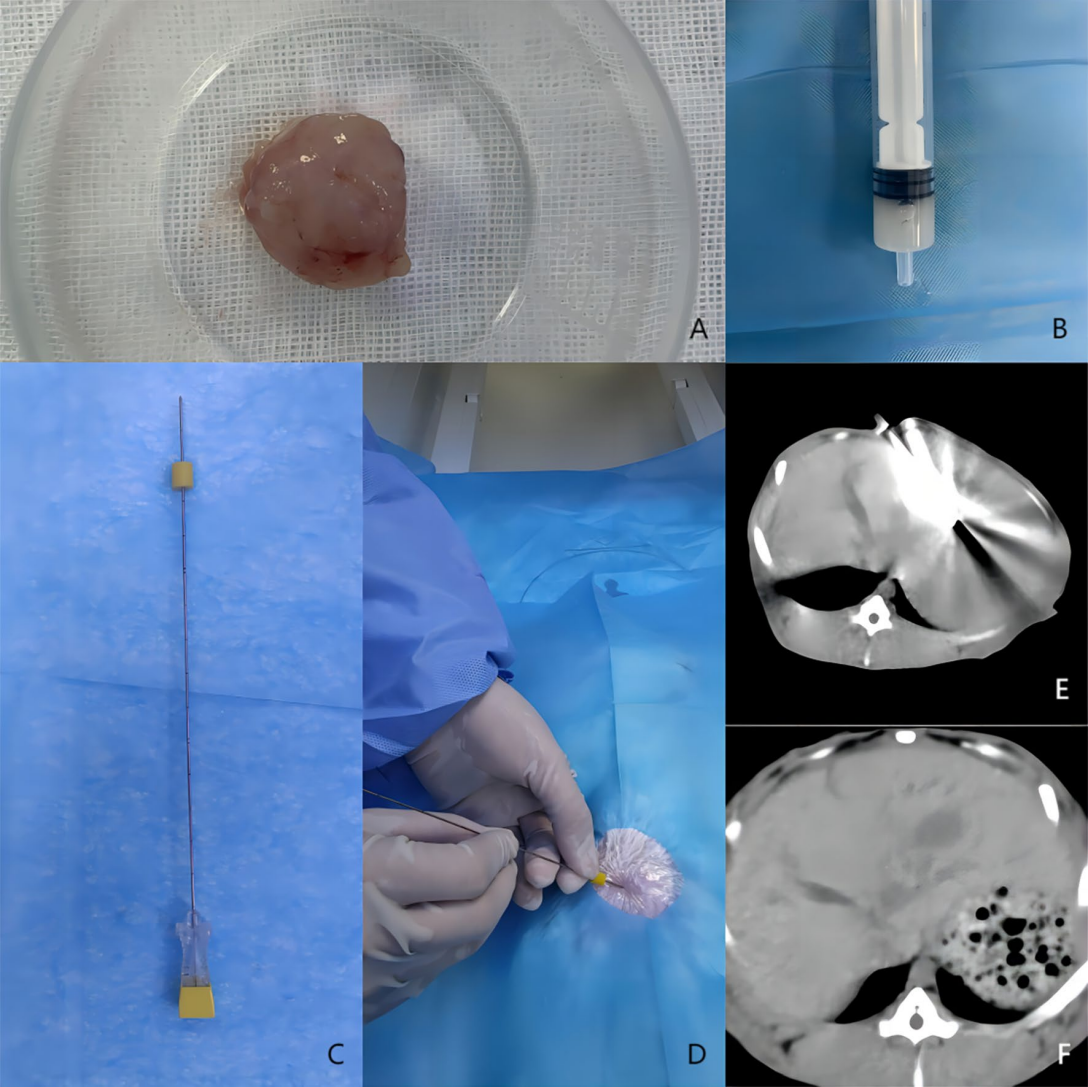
(A) Dissociated tumor tissue; (B) tumor tissue suspension prepared and loaded into a syringe; (C) puncture needle used; (D) after the skin was prepared, the puncture needle was introduced along the predetermined route; (E) the tip of the needle was placed in the predetermined implantation position in the liver; (F) CT scan before ablation, which shows the formation of a low-density lesion at the corresponding location within the liver.
